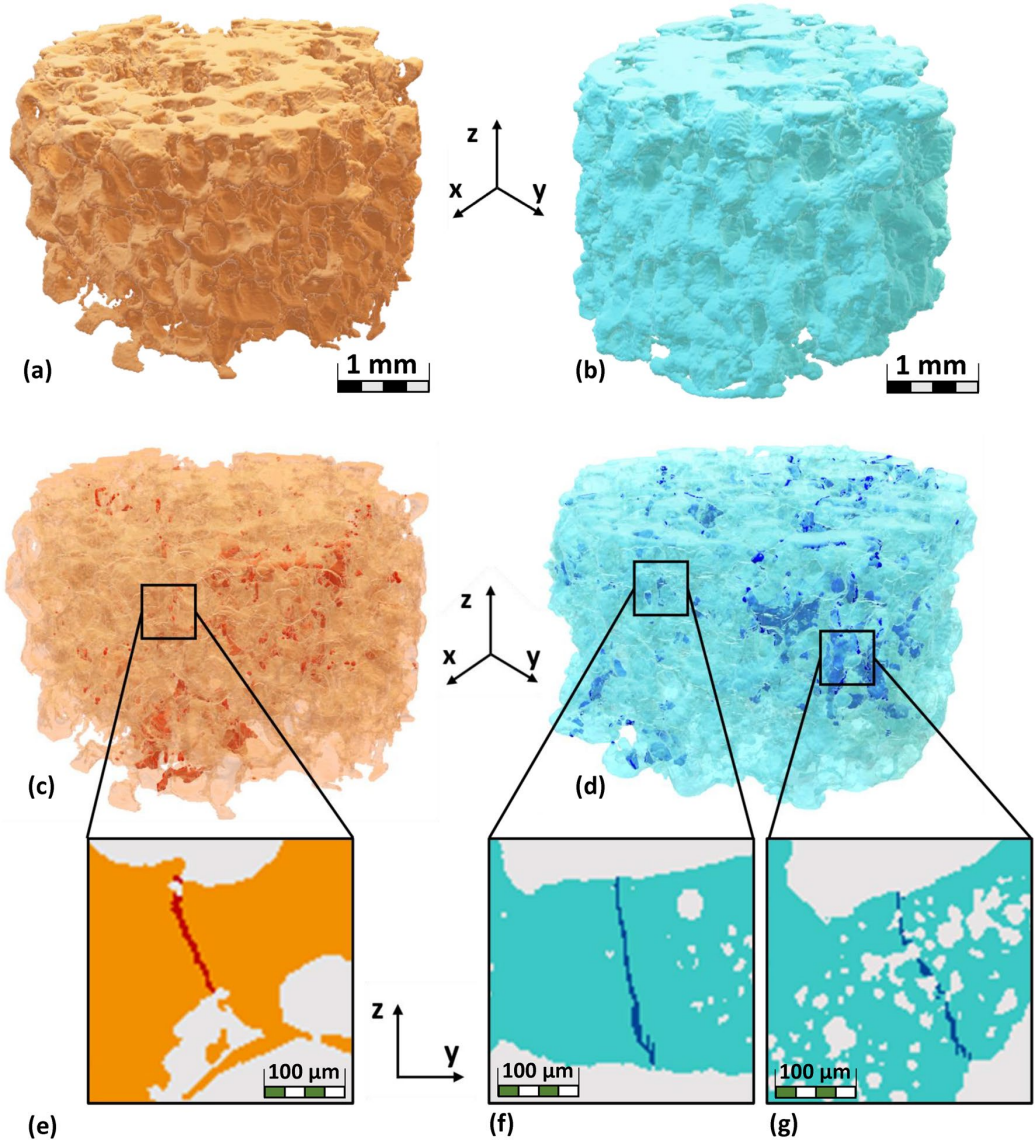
(a) $$\upmu$$CT reconstruction of a LT scaffold; (b) $$\upmu$$CT reconstruction of a HT scaffold; (c) LT scaffold highlighting the fractures due to compressive load; (d) HT scaffold highlighting the fractures due to compressive load; (e) fracture connecting large pores in LT scaffold; (f) fracture connecting large pores in HT scaffold; (g) fractures connecting small pores in HT scaffold.
